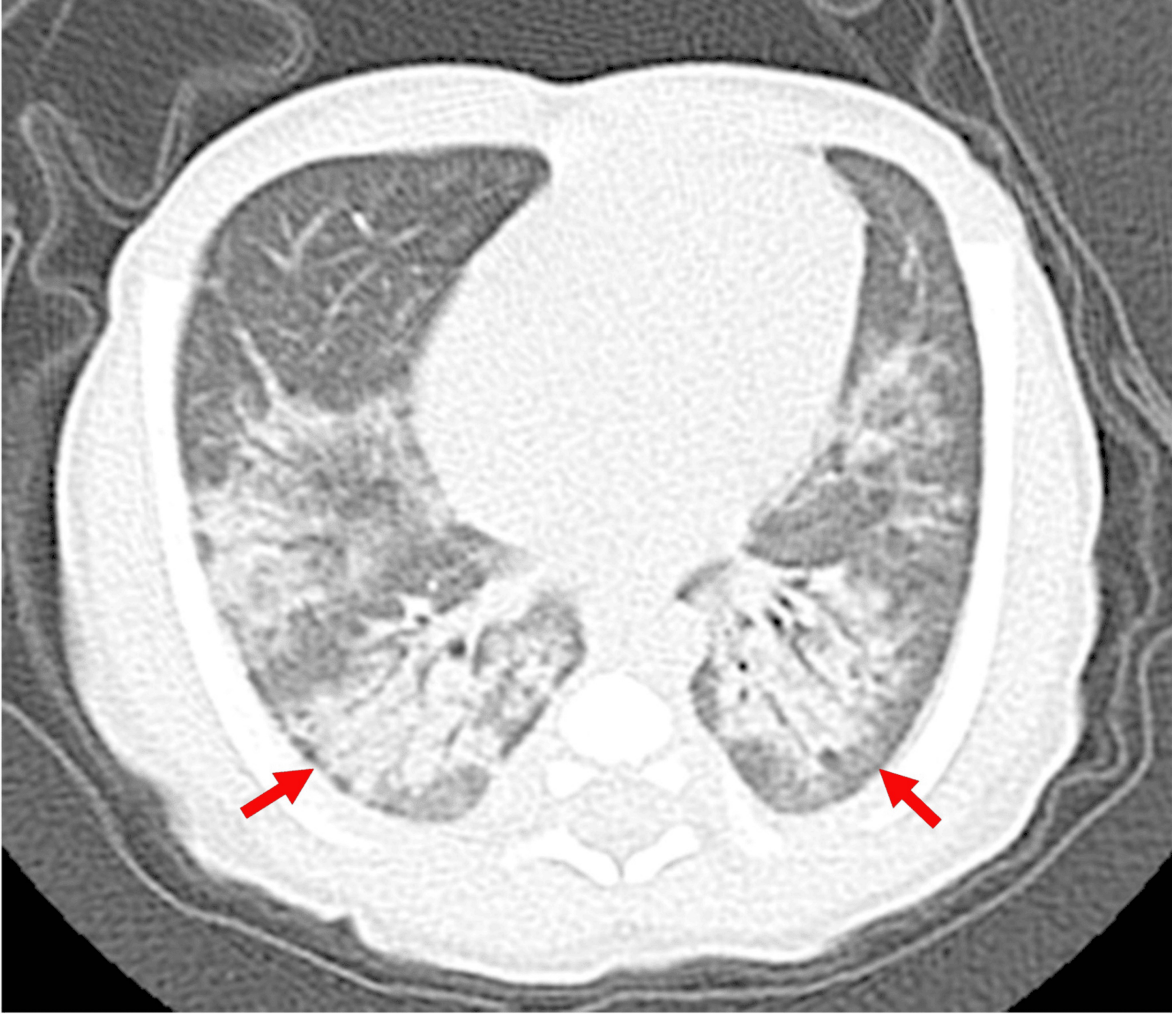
Chest computed tomography from day five Infiltrative shadows with air bronchograms are visible throughout the dorsal lung fields, suggesting pneumonia in these regions. The arrow indicates an area in which the subpleural area is spared from the infiltrative shadow. Ground-glass opacities are present in the surrounding tissue. Bronchial wall thickening was also observed.
